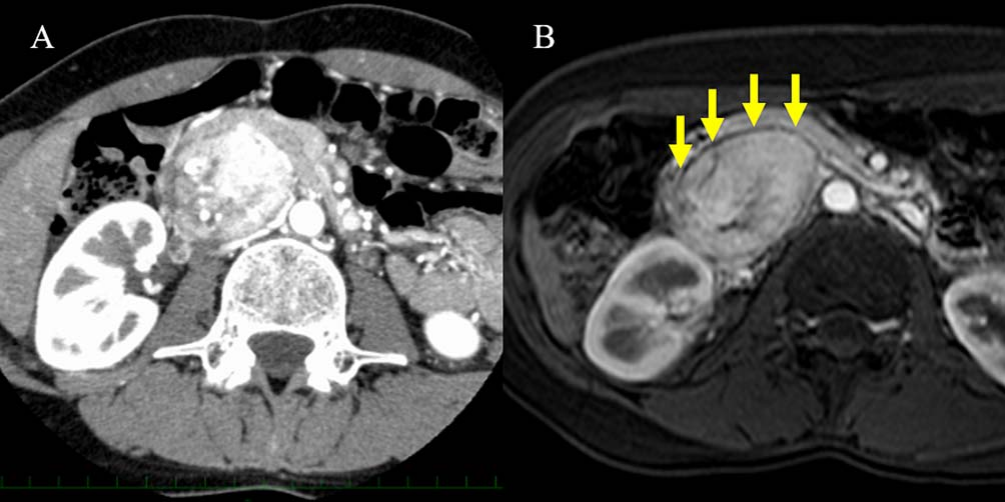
CT scan and MRI. (A) The hypervascular tumor was located dorsal to the pancreatic head in the arterial phase of contrast-enhanced CT scan. However, the outline of the tumor adjacent to the pancreas was somewhat unclear. (B) Fat-saturated T1-weighted MRI showing a clear plane between the tumor and pancreas.
